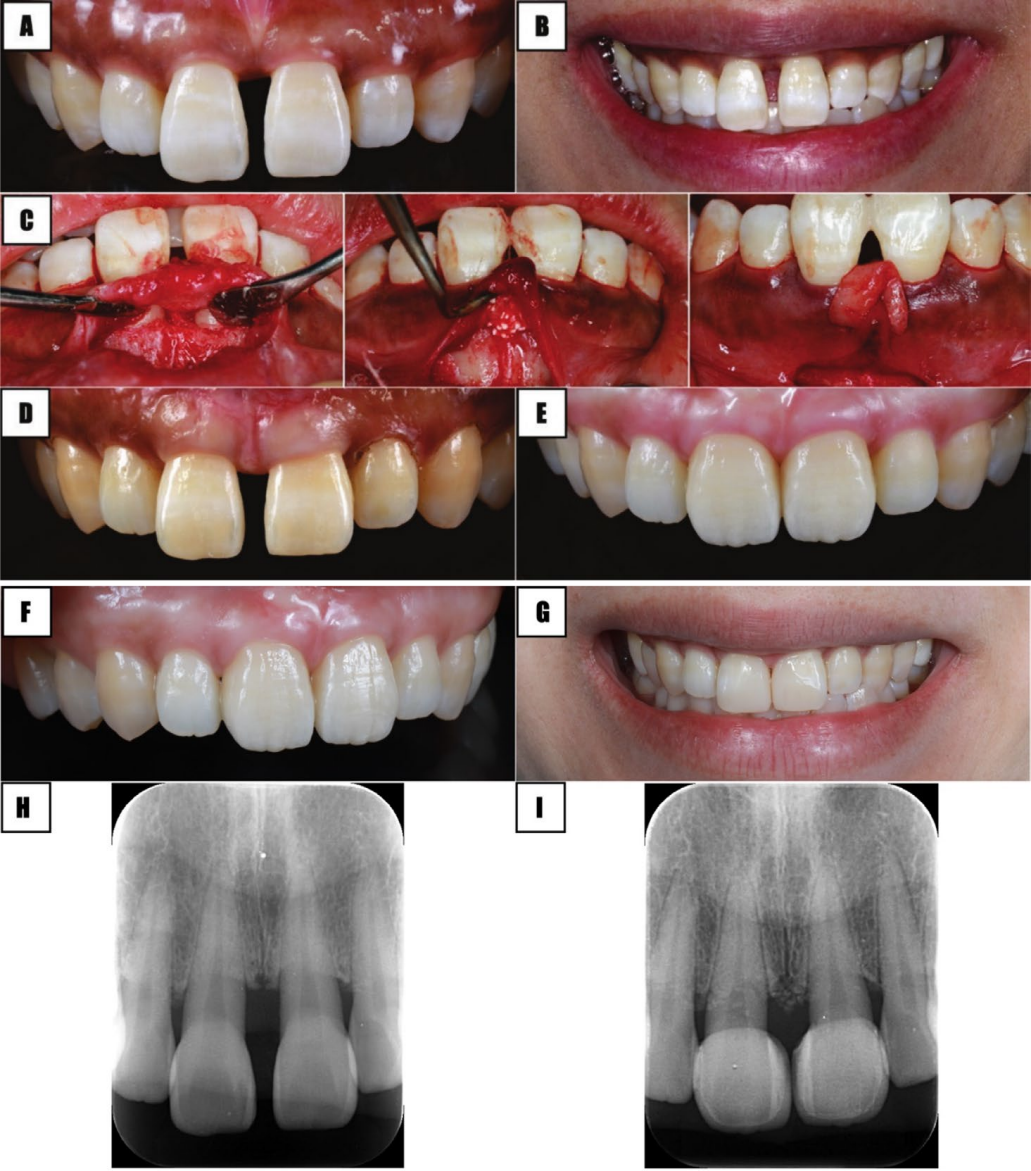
Scenario 3: healthy periodontal tissues—restoration and plastic surgery. (A and B) Initial intraoral condition, (C) periodontal surgery, (D) aspect after periodontal surgery, (E) frontal view after restorative treatment. (F) Veneer treatment and postoperative condition. (G) At 3 years post‐operation. (H) Initial periapical radiograph. (I) At 3 years follow up periapical radiograph.
